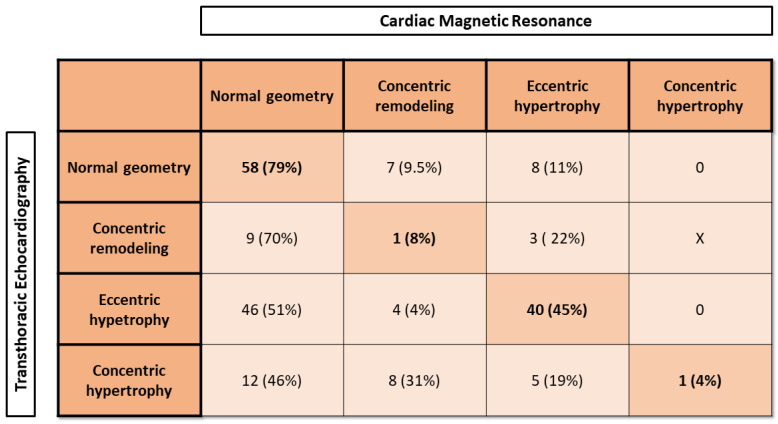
Left-ventricle remodeling type in transthoracic echocardiography and cardiac magnetic resonance.
